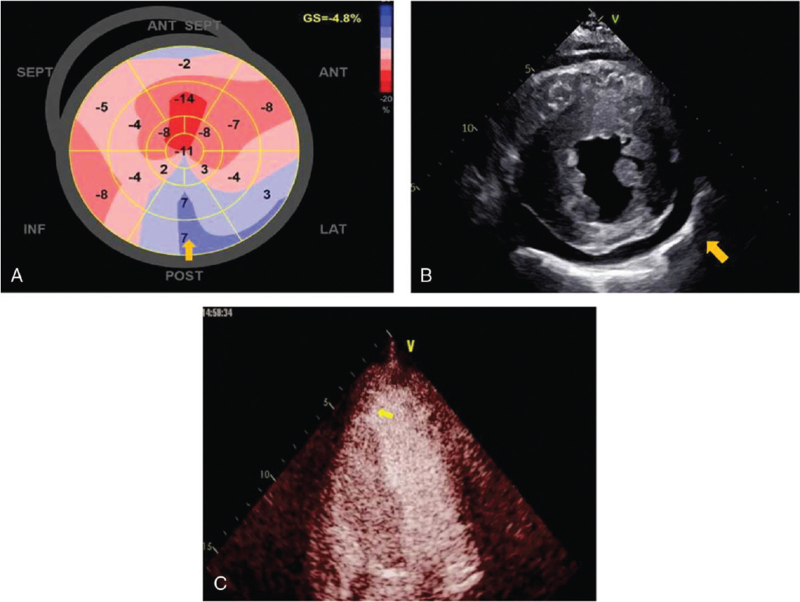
(A and B) Speckle tracking two-dimensional echocardiography strain analysis technology: left ventricular thrombosis, decreased movement of the inferior and posterior walls, and the basal segment of the posterior wall was locally fibrotic. (C) Myocardial contrast echocardiography: left ventricular thrombosis.
